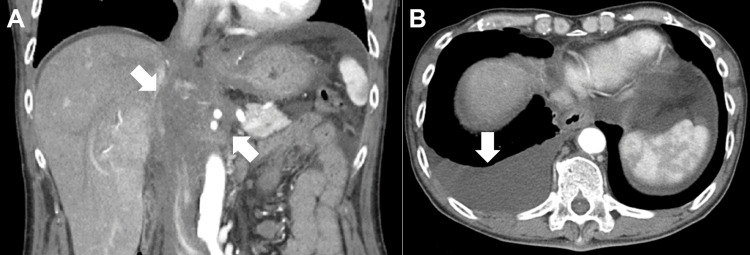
Computed tomography findings. An irregular mass (arrows) extending from the peri-esophagogastric junction to the retroperitoneum was identified (A). Right-sided pleural effusion was observed (B, arrow).
